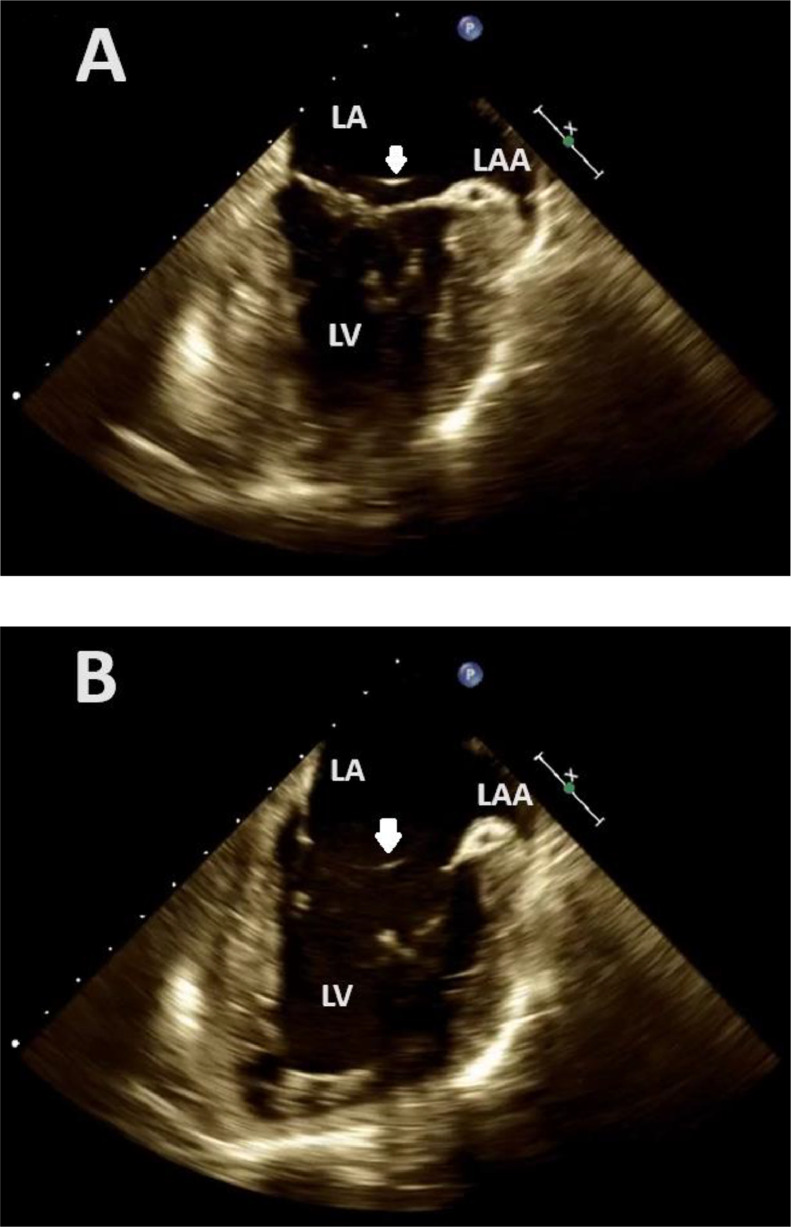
Two- and 3-dimensional transesophageal echocardiography showing a mid-esophageal commissural view during the systolic phase (A) and diastolic phase (B) demonstrate an echo dense structure (arrow) affixed to the left intra-atrial septum, extending across the mitral valve and connecting to the orifice of the left atrial appendage. LA, Left atrium; LV, Left ventricle; LAA, Left atrial appendage.
